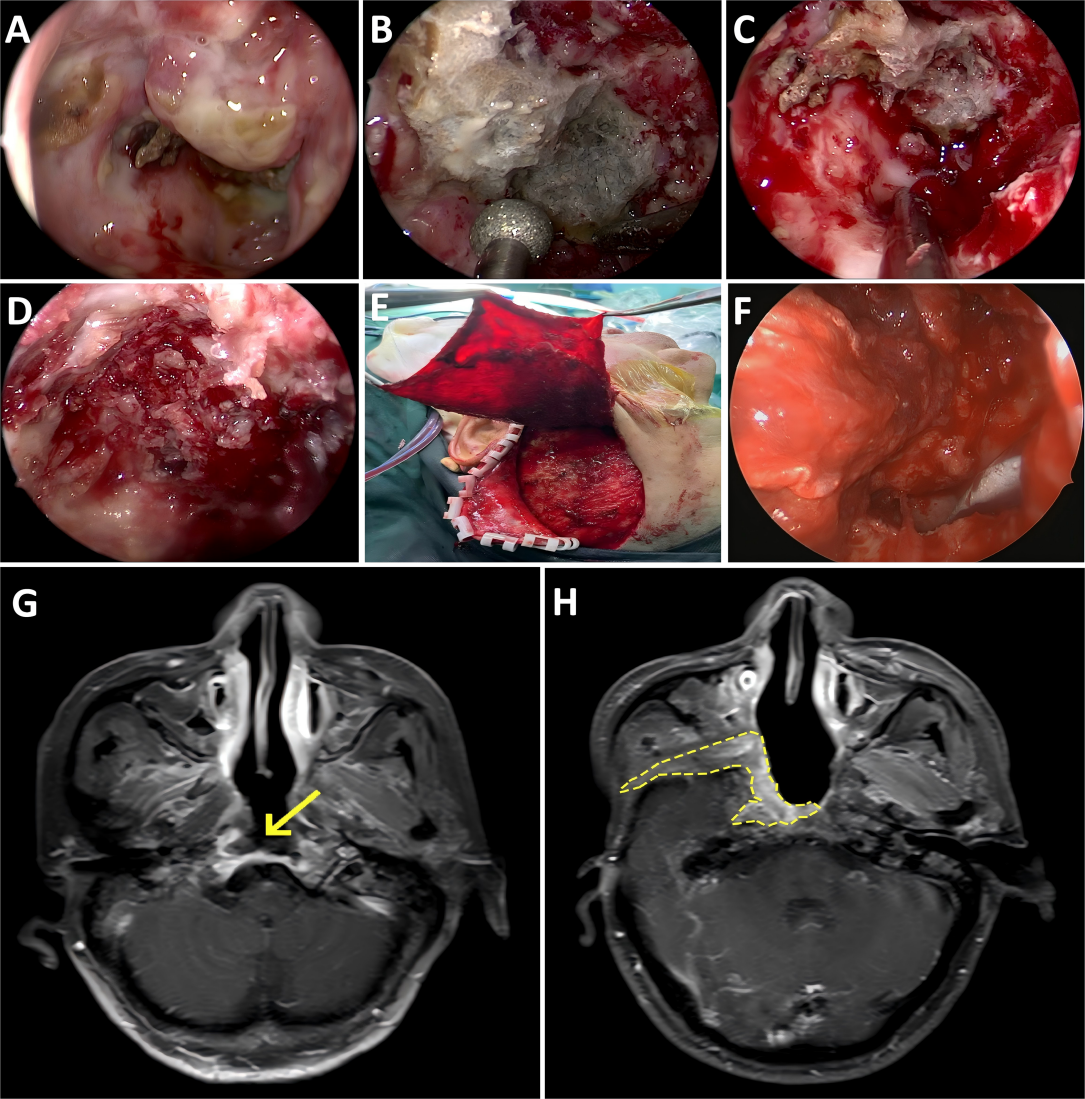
Surgical procedures and magnetic resonance images. (A) Necrotic ulcers in the nasopharynx. (B, C) Removal of bone necrosis at the base of the skull using a drill. (D) Fresh bone at the base of the skull. (E) Temporal muscle flap. (F) Repair of the skull base wound using a temporal muscle flap. (G) Preoperative image: The arrow points to the nasopharyngeal ulcer accompanied by skull base necrosis. (H) Postoperative image: The yellow dotted line indicates that the temporal muscle flap had survived.
